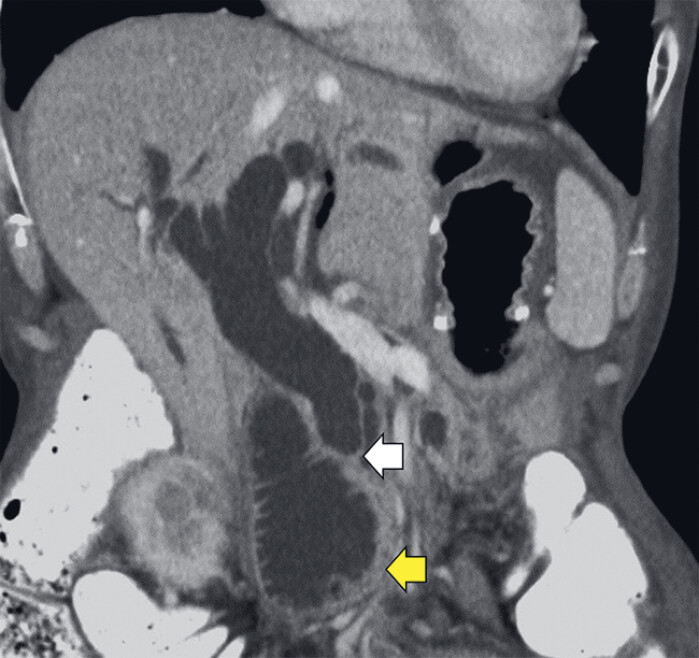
Contrast-enhanced computed tomography (CE-CT; coronal view) demonstrates peritoneal dissemination-induced stenosis of the distal bile duct (white arrow) and the third portion of the duodenum (yellow arrow), with dilatation of the bile duct and the afferent loop. The dilated afferent loop is situated at a considerable distance from the remnant stomach.
